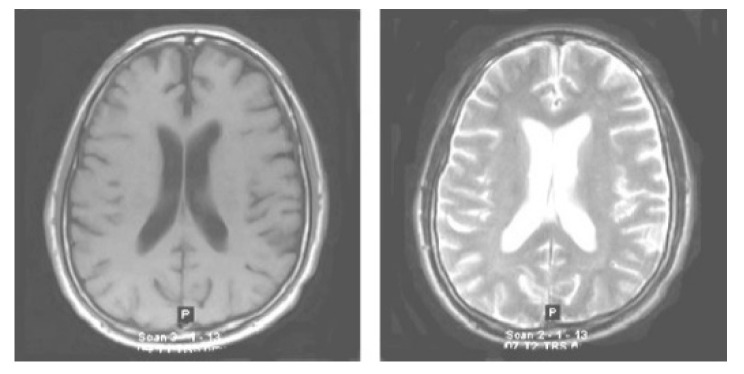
Original normal brain T1-weighted and T2-weighted MRI images.
